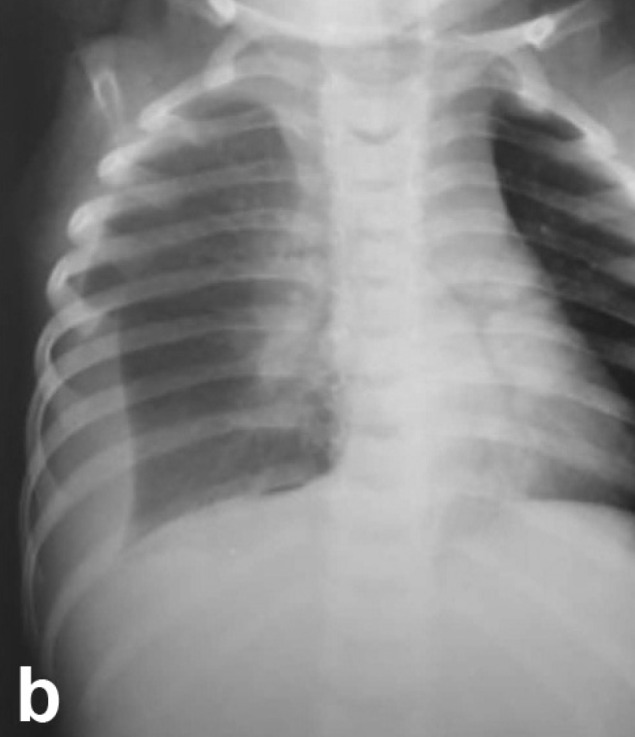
Chest X-ray on day 5 of admission, demonstrating right pleural
